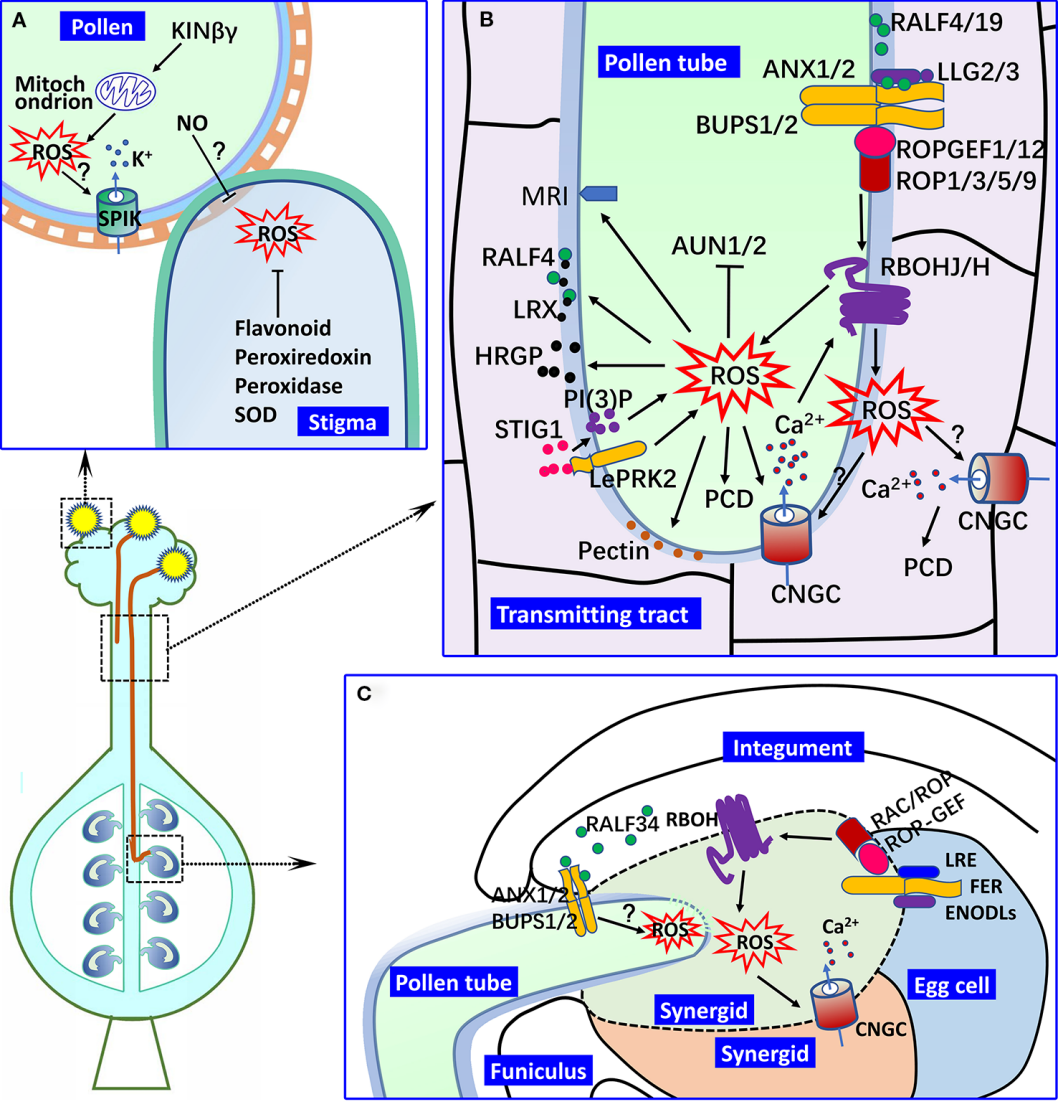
ROS in male–female interactions. (A) Pollen hydration and germination on the stigma. In *Arabidopsis* pollen, KINβγ mediates the biogenesis of mitochondrion and ROS levels, which regulate pollen hydration and germination on the stigma. In this process, the expression of *SPIK* might be regulated by ROS signaling, by which ROS signaling mediates K^+^ transport and pollen hydration on the stigma. ROS accumulation is found in receptive stigma, which is important for pollen attachment, but its decrease is required for the germination of compatible pollen. The ROS levels of the stigma are controlled by various oxidoreductase and flavonoids and could also be regulated by NO from the pollen. (B) Pollen tube growth in the pistil. NAD(P)H oxidase RBOHH/J-mediated apoplastic ROS production in the growing tip of the pollen tube is important for pollen tube integrity and growth in the pistil by regulating the activities of calcium channels (such as the CNGCs), the secretion of HRGPs (such as LRXs), and the metabolism of wall materials (such as pectin and callose). A type one protein phosphatase (AUN1/2) and plasma membrane-localized receptor-like cytoplasmic kinase MRI also function downstream of ROS signaling in pollen tube growth. The RALF-LLG-BUPS-ANX receptor–ligand interaction is involved in the active regulation of RBOHs and ROS generation, which is mediated by ROPGEFs and ROPs. Pistil-derived STIG1 induces the ROS production of the growing pollen tube in the transmitting tract mediated by LePRK2 and PI(3)P. Apoplastic ROS of the pollen tube might induce PCD of the transmitting tract by mediating CNGC activity and Ca^2+^ signaling. (C) Pollen tube reception in the female gametophyte. Pollen tube rupture in the synergid is controlled by ROS from RBOHs in the female gametophyte. LRE and ENODLs serve as the co-receptors of FER to regulate the activity of RBOHs and ROS generation in the synergid, and RAC/ROP might mediate this process. RALF34 primarily derived from the inner integument controls the pollen tube rupture in degenerated synergid by binding to the BUPS/ANX receptor complex in the pollen tube, during which ROS might act downstream of the BUPS/ANX receptor complex. (? indicates the putative regulation.).
